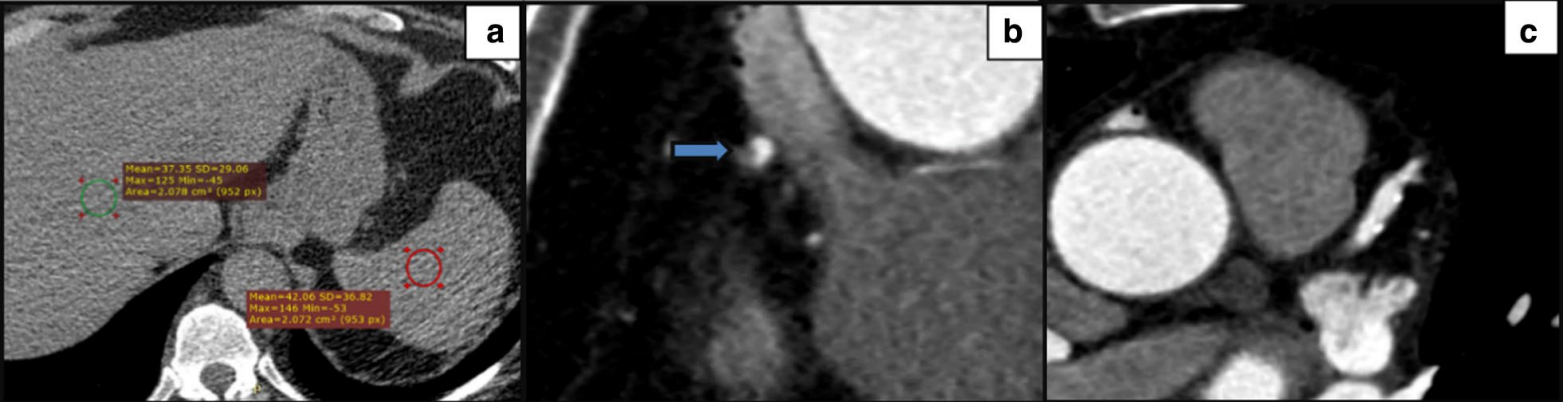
Diabetic male patient 67 years old. a Axial non-enhanced CT image demonstrates attenuation measurements of the liver and spleen shows a diffuse fat accumulation in the liver, with a mean liver attenuation of 37.3 HU and a mean spleen attenuation of 42.5 HU. b Coronary CT angiography images shows a non-calcified plaque demonstrates a napkin-ring sign (arrow) with a central low-attenuation area, surrounded by a peripheral rim of higher attenuation and c Coronary CT angiography images shows a plaque with spotty calcium
